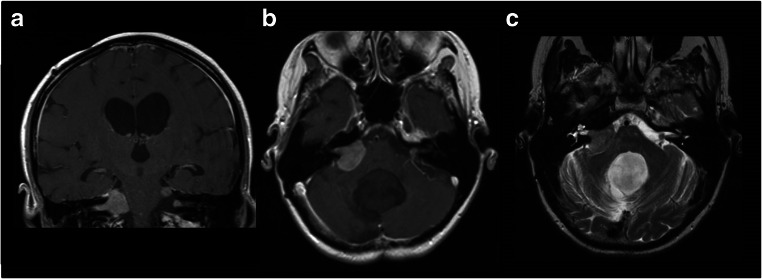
Coronal (A) and axial (B + C) MRI of type 1 choroid plexus papilloma: tumor portions are present in the cerebellopontine angle, without any tumor portions in the cerebellomedullary angle (superior to the line between the pons and medullar oblongata) (this patient had also drop metastases in the contralateral cerebellopontine angle)
